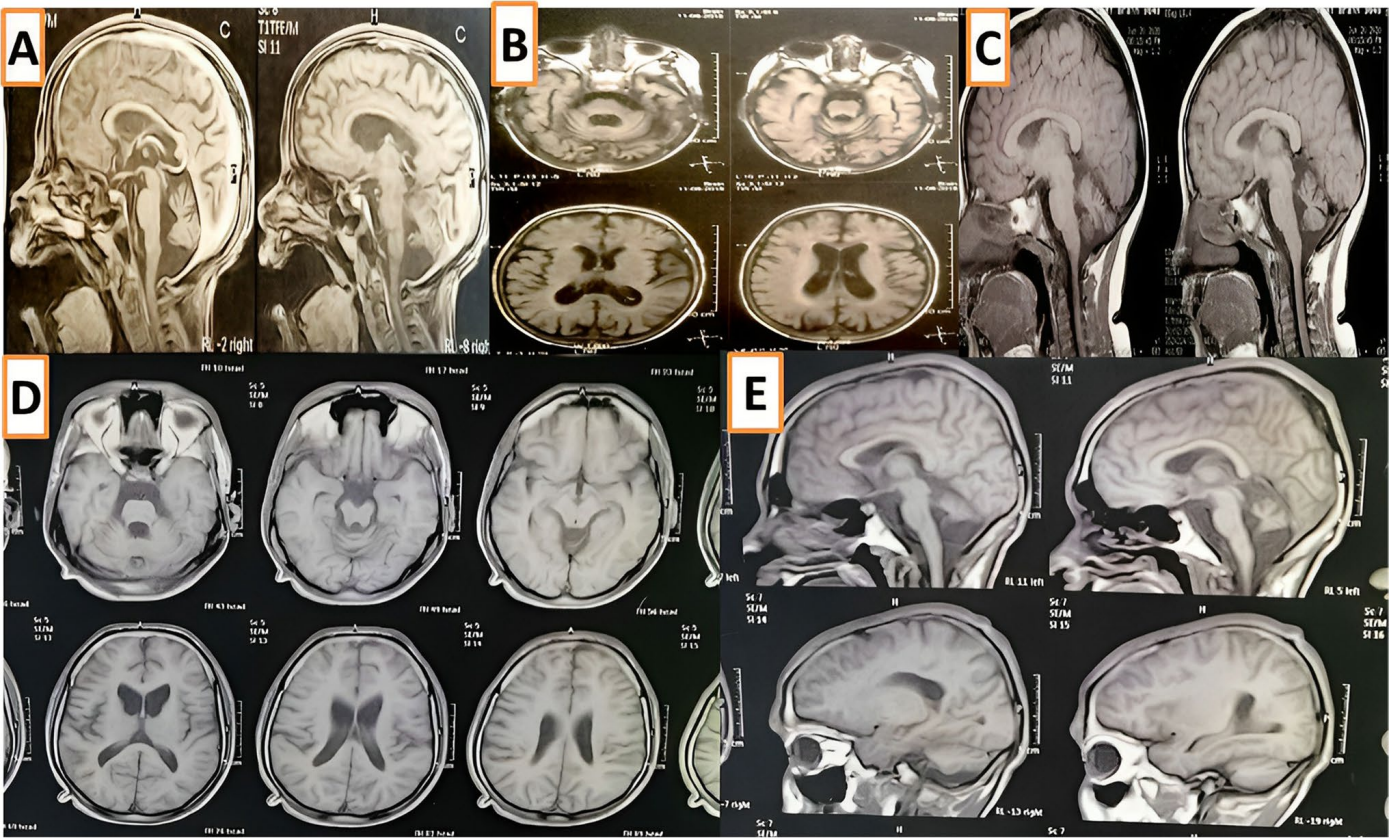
Brain MRI findings of the studied patients. P1 (A, B) cerebral and cerebellar atrophy and hypoplastic corpus callosum; P2 (C, D) cerebellar vermis hypoplasia; P3 (E) cerebellar atrophy
